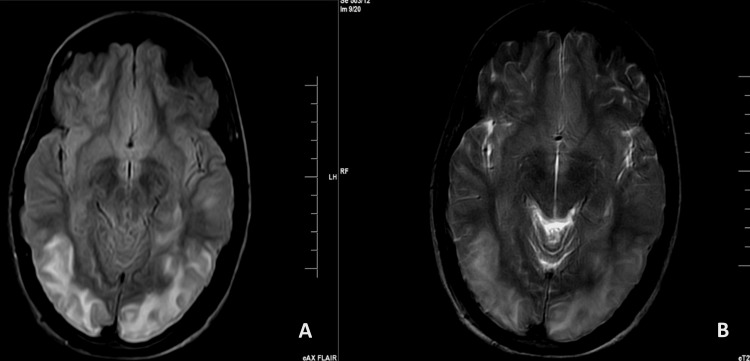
FLAIR MRI (A) and T2WI MRI (B) image axial section showing hyperintense areas in the bilateral temporo-occipital regions FLAIR: Fluid-attenuated inversion recovery; T2WI: T2 weighted image
